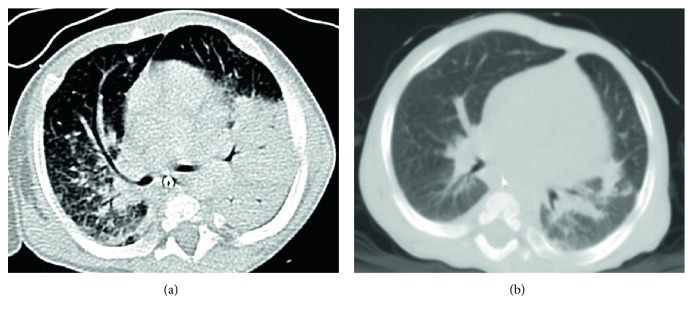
CT scan of a possible invasive fungal infection in a 5-month-old XCGD patient (a) pre-HSCT and (b) after HSCT. A progressive improvement of the areas of consolidation in the left and right lungs, especially for the right lobes, is observed. Residual pulmonary lesions are visible in the left and right inferior lobe.
